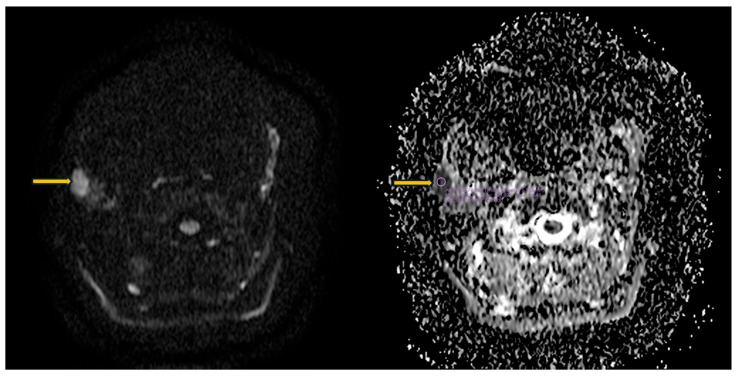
MRI diffusion-weighted imaging (DWI) and ADC map of the parotid gland. Axial images show a right parotid nodular lesion with restricted diffusion (arrows). The purple circle indicates the region of interest (ROI) used for calculation of the apparent diffusion coefficient (ADC), which is markedly reduced (0.460 × 10^−3^ mm^2^/s), a pattern suspicious for salivary gland lymphoma in the context of Sjögren’s syndrome.
